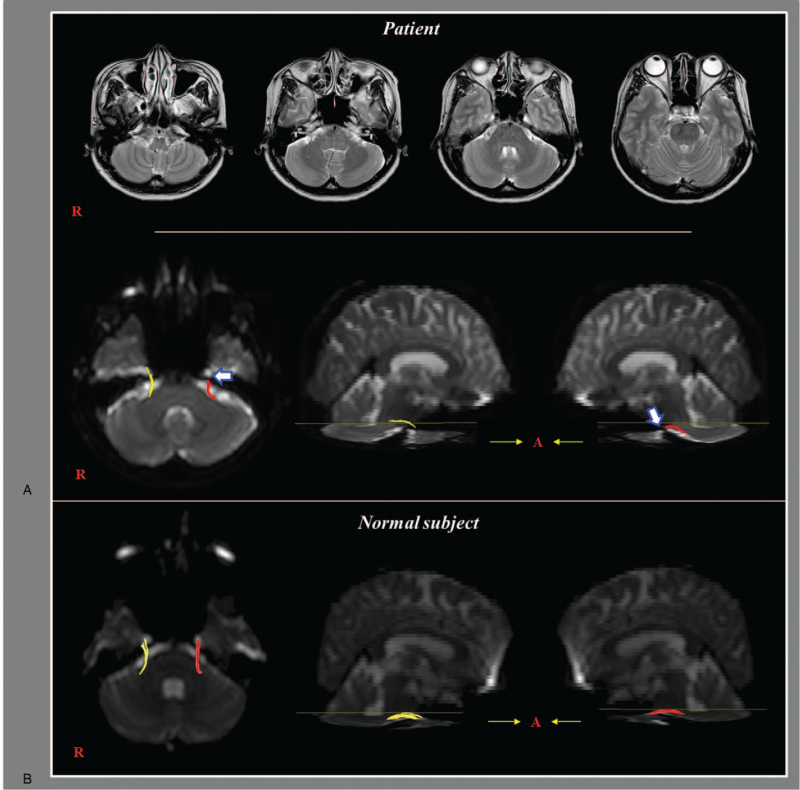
(A) T2-weighted brain magnetic resonance (MR) images obtained 7 days after whiplash onset show no abnormality (upper row). Results of diffusion tensor tractography: the left trigeminal nerve is discontinued (arrow) (lower row). (B) Results of diffusion tensor tractography for a control subject (53-year old female).
